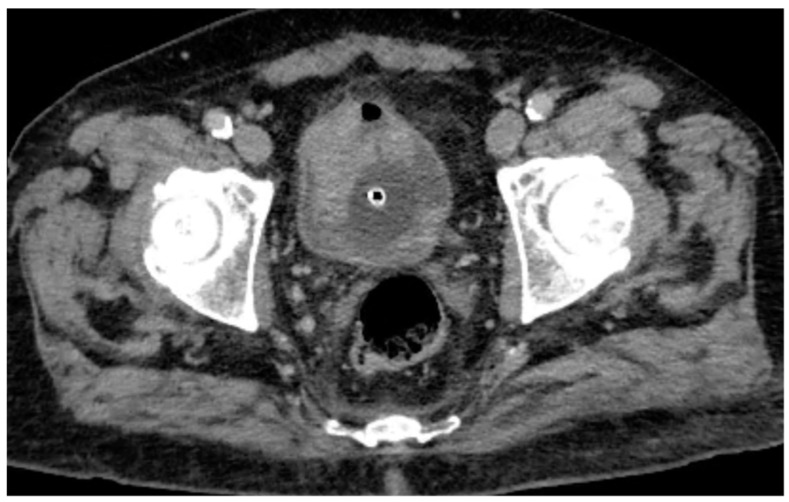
Venous phase CT scan confirms the previously described findings, showing a lesion involving the anterior and left posterolateral bladder wall, with an associated hyperdense intraluminal component.
